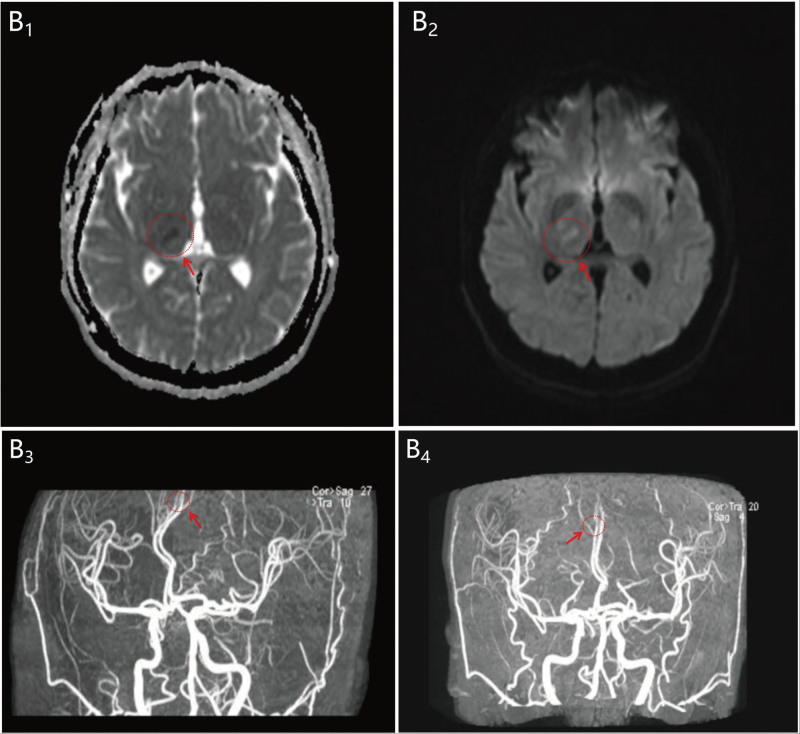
Magnetic Resonance Imaging (MRI). (B_1_ and B_2_) An MRI examination of the head displayed the right thalamus was speckled with abnormal signal, a slightly high signal on DWI, and a low signal on ADC. (B_3_ and B_4_) Intracranial magnetic resonance angiography excluded intracranial large vessel occlusion and showed a right anterior cerebral artery (A4 segment) stenosis.
